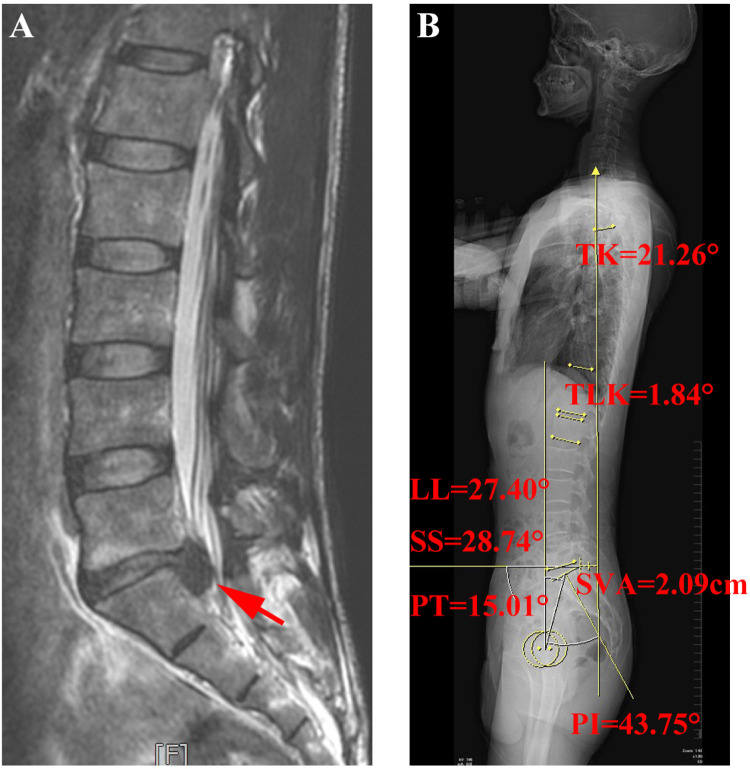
MRI imaging and spinal-pelvic parameters of a patient diagnosed with HDM-LDH. (A) Sagittal MRI. (B) Measured spinal-pelvic parameters. White arrows indicate the location of disc herniation. Patient information: male, 37 years old; diagnosed with L5–S1 disc herniation; preoperative MRI reveals a high-grade downward migration of the herniated fragment at the L5–S1 level. PI, pelvic incidence; SS, sacral slope; LL, lumbar lordosis; TK, thoracic kyphosis; PT, pelvic tilt; SVA, sagittal vertical axis; TLK, thoracolumbar kyphosis.
